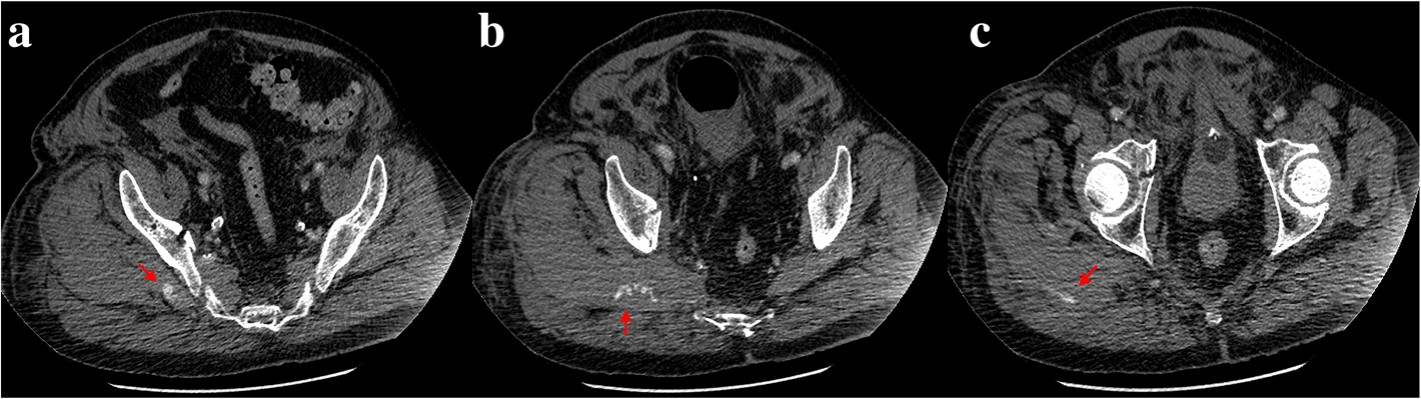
a-c An 83-year-old road traffic collision (RTC) male patient admitted with significant pelvic fracture and haemodynamic instability (on Warfarin). Series of axial images (a-c) from an urgent arterial phase CT scan (1 mm slice thickness) showing extensive right gluteus medius hematoma (14 × 4.5 cm) with high-density foci of contrast extravasation spreading (red arrows) inferolateral to an acutely fractured right iliac bone, see online Additional file 2
